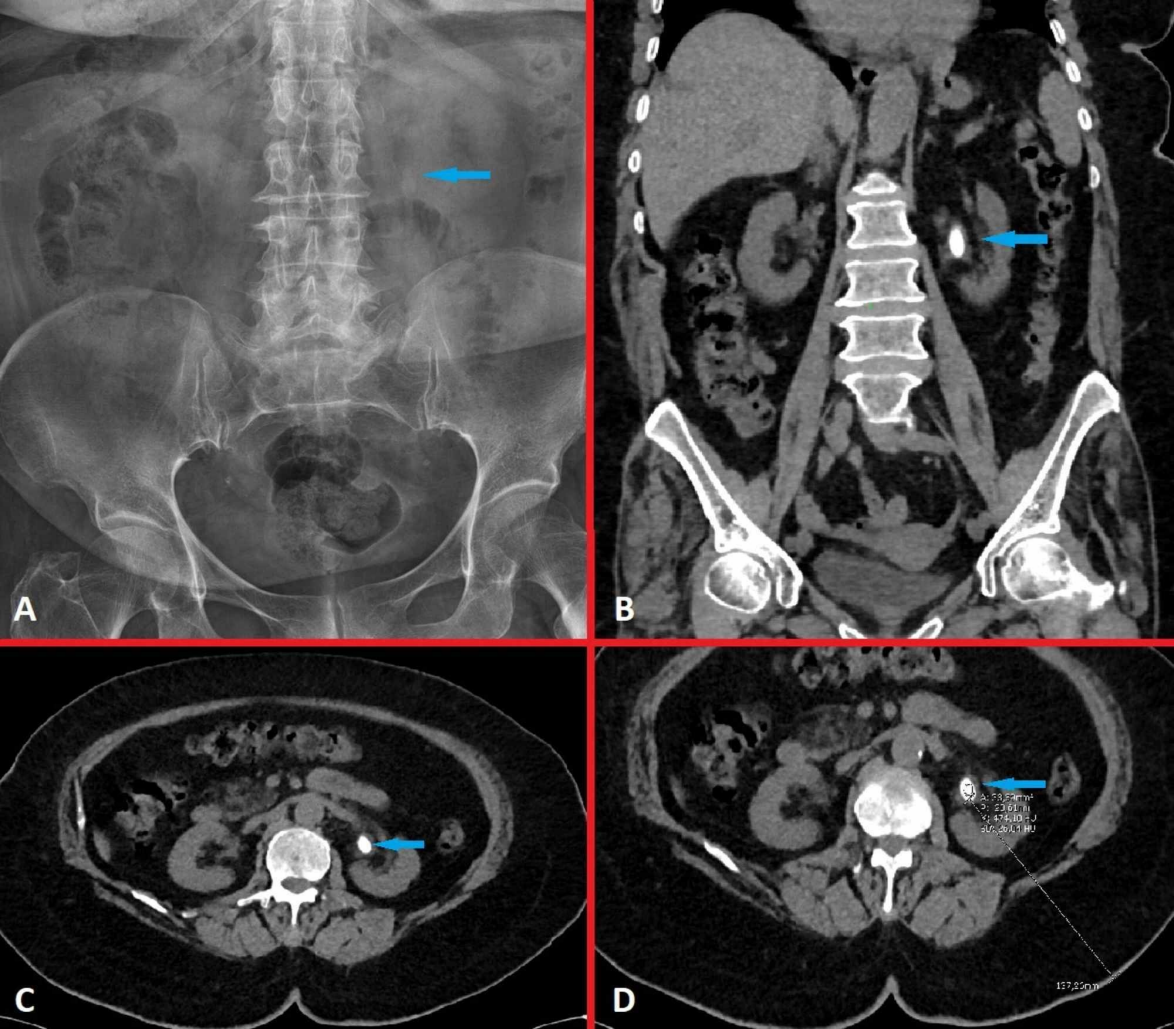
Radiological imaging before ESWL treatment A 10-mm sized semi-opaque stone in the left renal pelvis on KUB X-ray (A), in coronal (B) and in transverse cross-sections (C) on NCCT. Stone density (440 Hounsfield units) and skin to stone distance (13 cm) were shown in transverse cross-section on NCCT (D). ESWL: extracorporeal shock wave lithotripsy, KUB: kidney-ureter-bladder, NCCT: non-contrast computed tomography.
